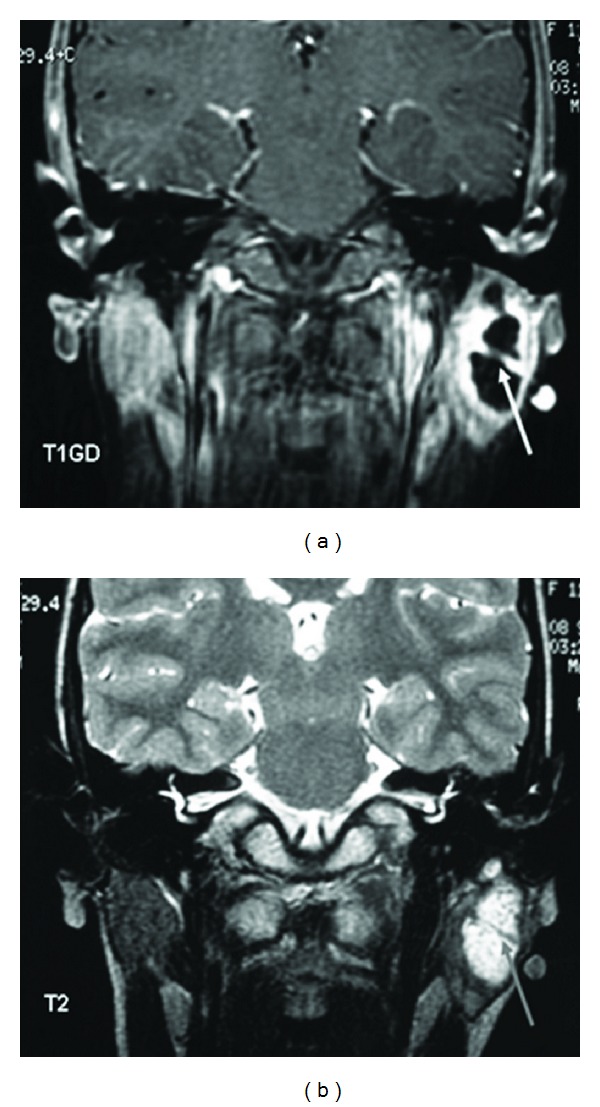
Coronal MR T1-contrast enhanced and T2-weighted images demonstrating the lesion in the left parotid gland (arrows: cyst with internal septum).
